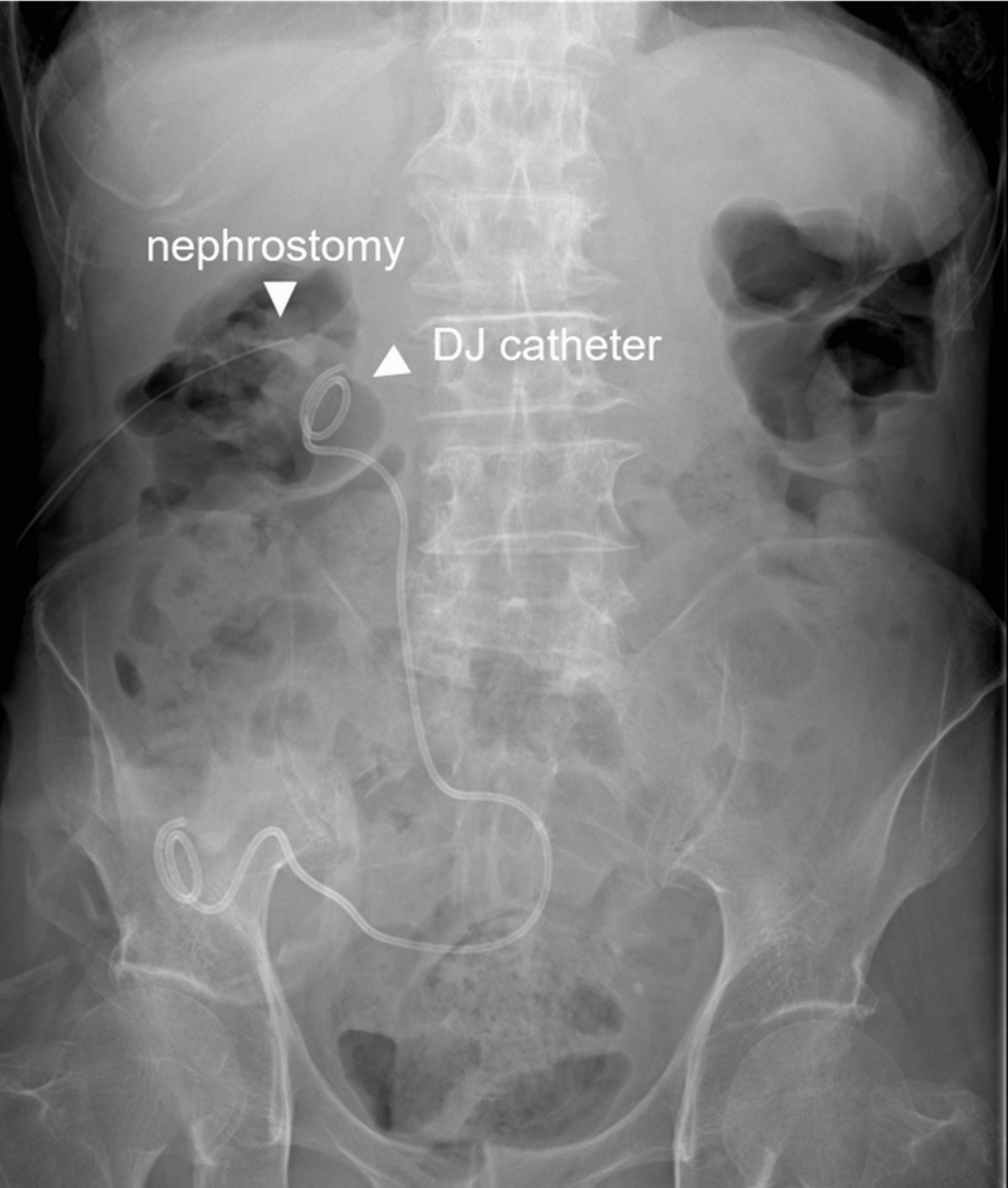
A plain abdominal radiograph taken on admission shows a right nephrostomy tube and a right double-J (DJ) catheter in place
